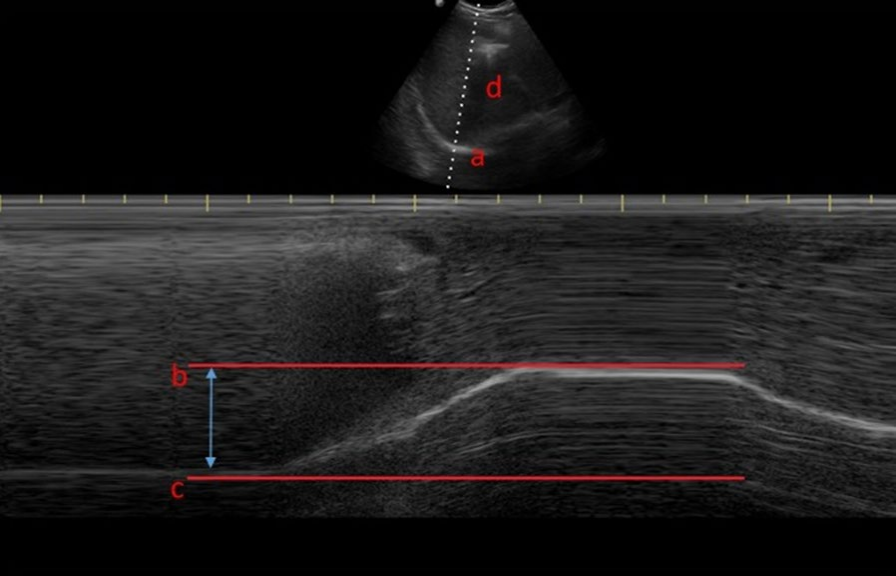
Diaphragm excursion as assessed via M-mode ultrasonography, where a is the diaphragm, b is at the end of a deep inspiratory effort, c is at end expiration and d is the liver
